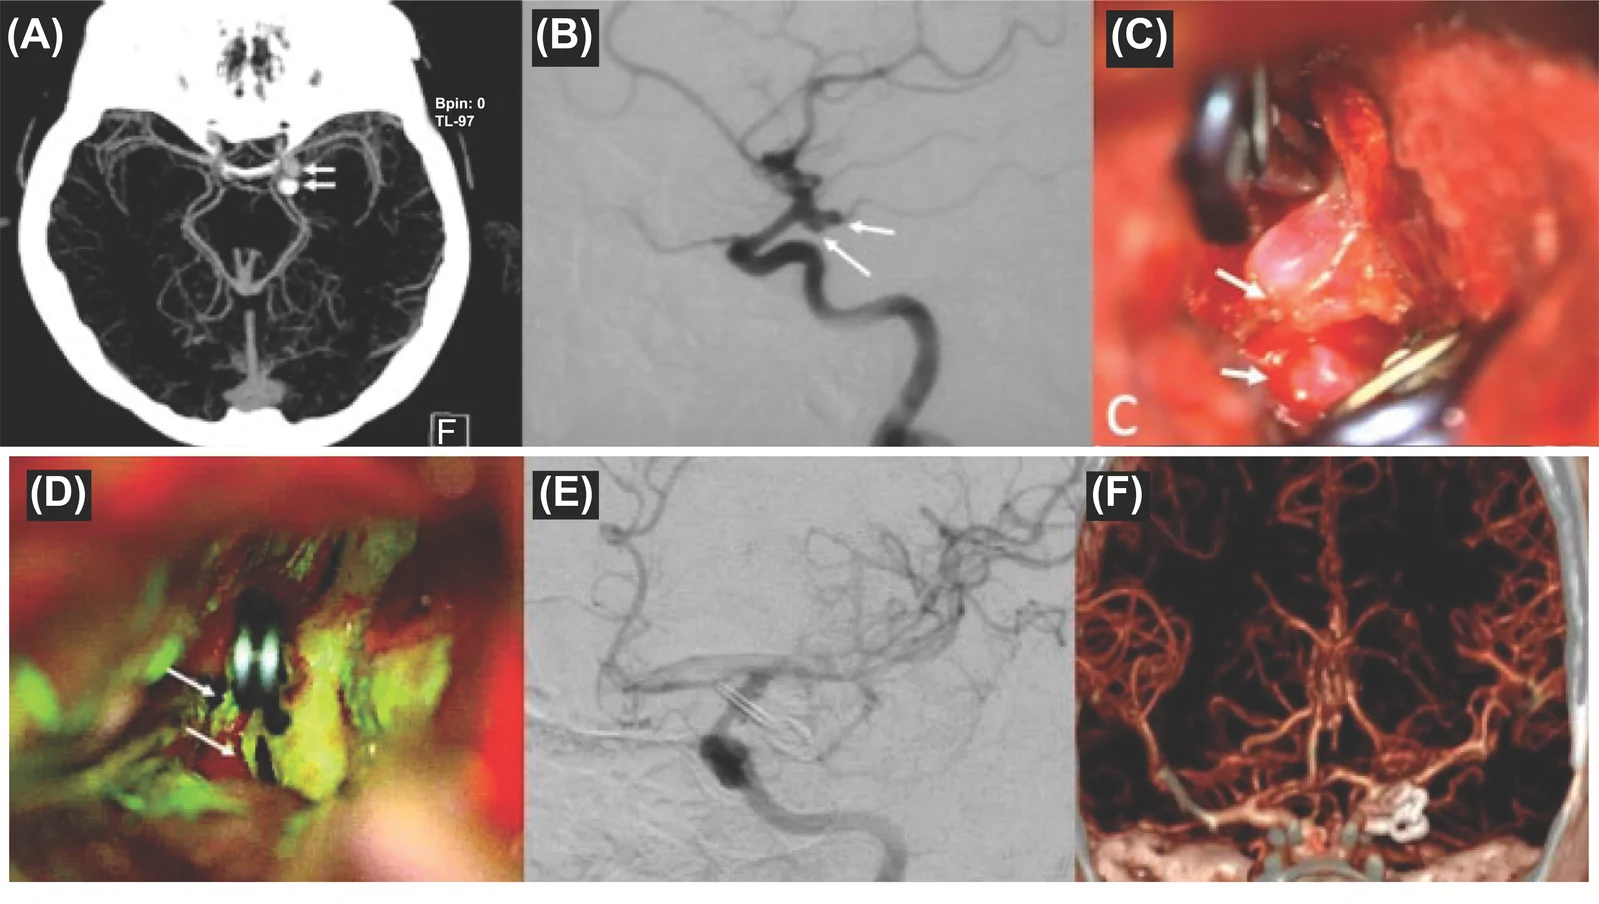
Case 2. (A) Axial CTA reconstruction showed a bilobulated aneurysmatic lesion on the left internal carotid artery (ICA). (B) DSA images confirm kissing aneurysms located at the PComA and AChA segments of the left internal carotid artery (ICA). (C) Intraoperative photograph displays both aneurysms dissected under temporary ICA clipping. (D) Fluorescein videoangiography confirms patency of the PComA and AChA (arrows). (E) Postoperative DSA shows complete occlusion of the aneurysms with patency of the parental vessel. (F) CTA confirms complete clipping of both aneurysms and adequate position of the clips.
